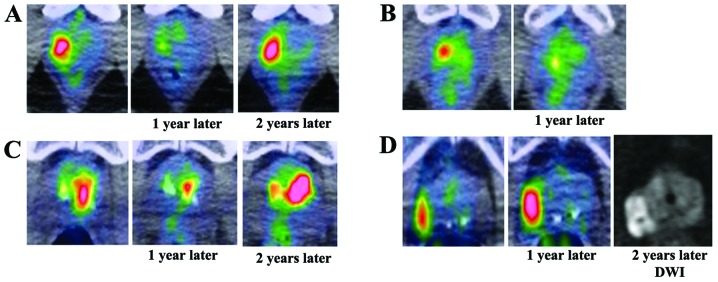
Reproducible FDG uptake. (A–C) All 3 cases were diagnosed as chronic inflammatory change such as prostatitis. Although SUVmax was different as assessed each year, the FDG uptake tended to exist in the same area. (D) One case was diagnosed as adenocarcinoma 2 years following the initial PET/CT scan. During those 2 years, biopsies were performed 3 times. DWI, diffusion weighted image.
